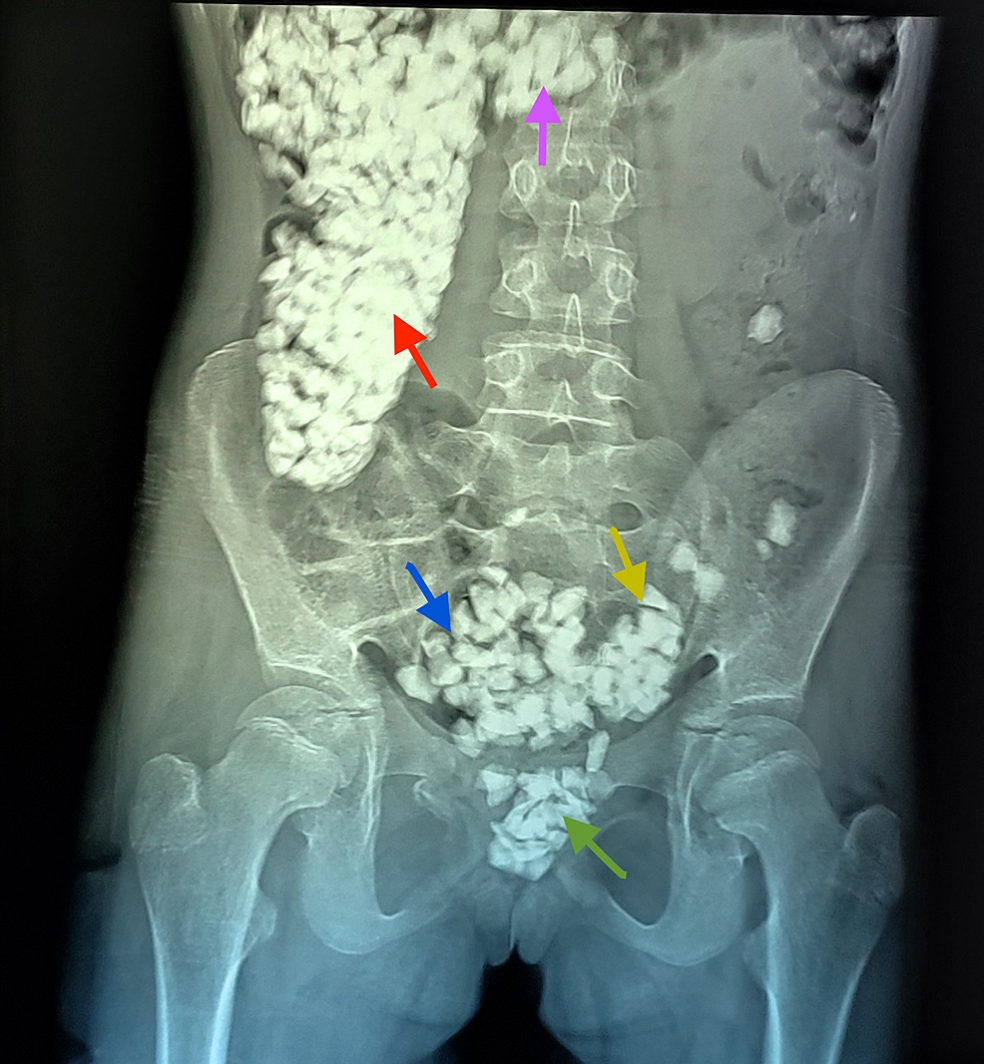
First abdominal X-ray of the patient taken in the emergency department. Plain abdominal radiograph (frontal projection) showing multiple radiopaque densities of variable sizes in the ascending (red arrow), proximal transverse (purple arrow), distal descending (yellow arrow), and sigmoid colon (blue arrow) along with the rectum (green arrow).
